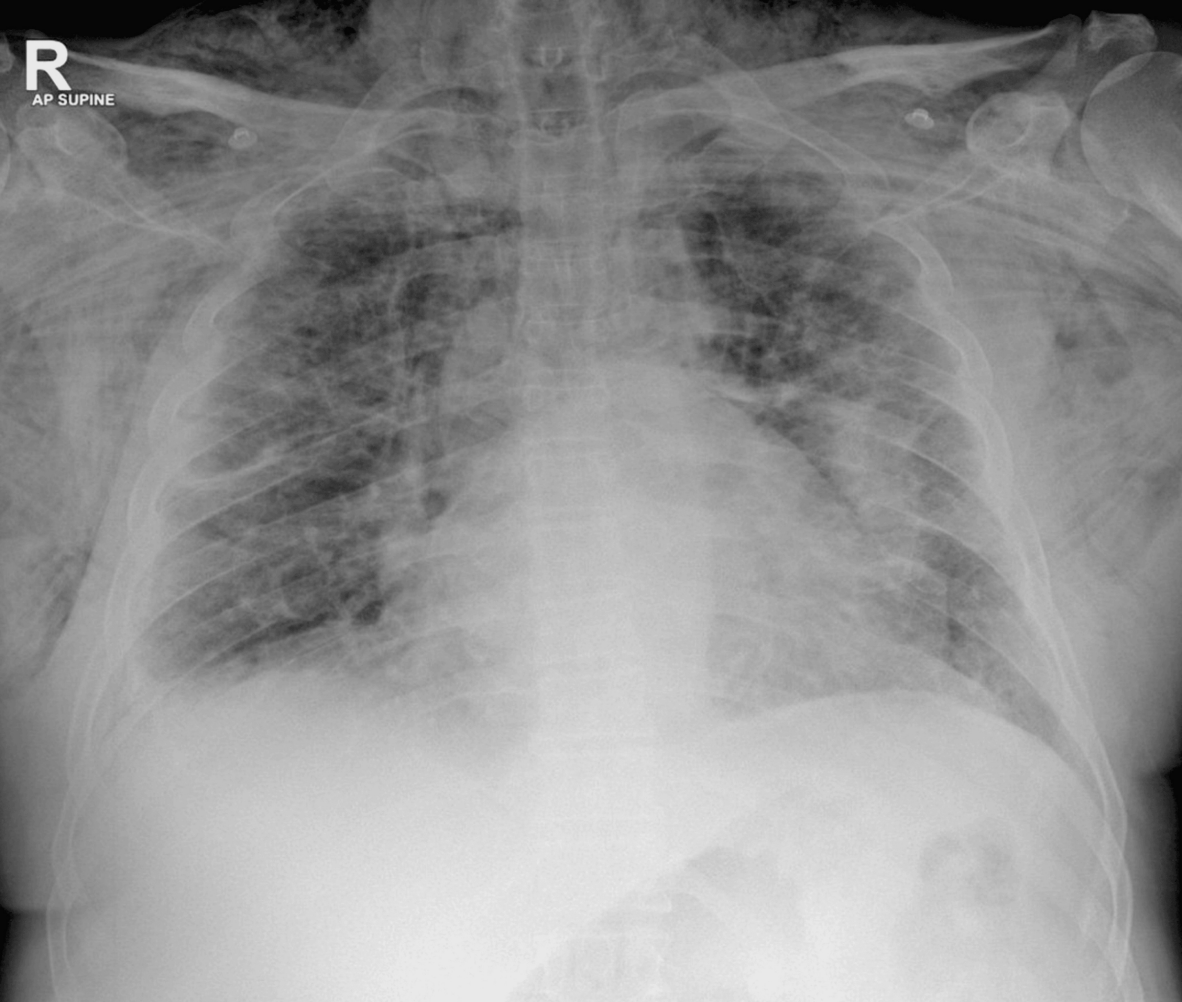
Chest X-ray (AP supine view) demonstrating diffuse subcutaneous emphysema with radiolucent streaks over the thoracic wall.
